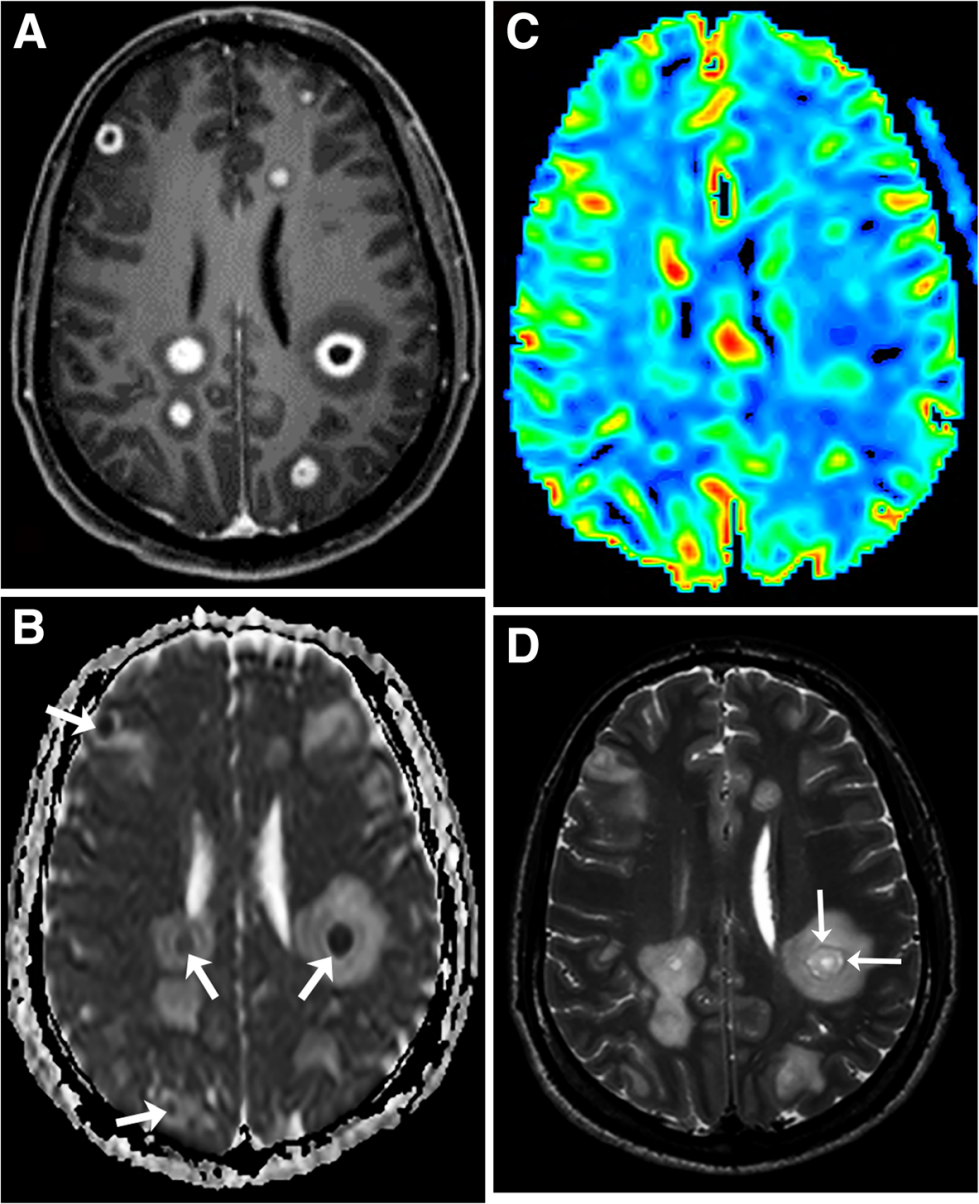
Streptococcus brain abscesses. T1-weighted image with gadolinium (a) demonstrates multiple lesions with rim enhancement and surrounding vasogenic edema. The central core of the lesions restrict strongly (arrows) on the ADC map (b). The rims are not restricted. CBV map (c) shows mild elevated perfusion in the lesions, but no high perfusion in the surrounding area. Note the T2 hypointense rim (arrows) (d)
